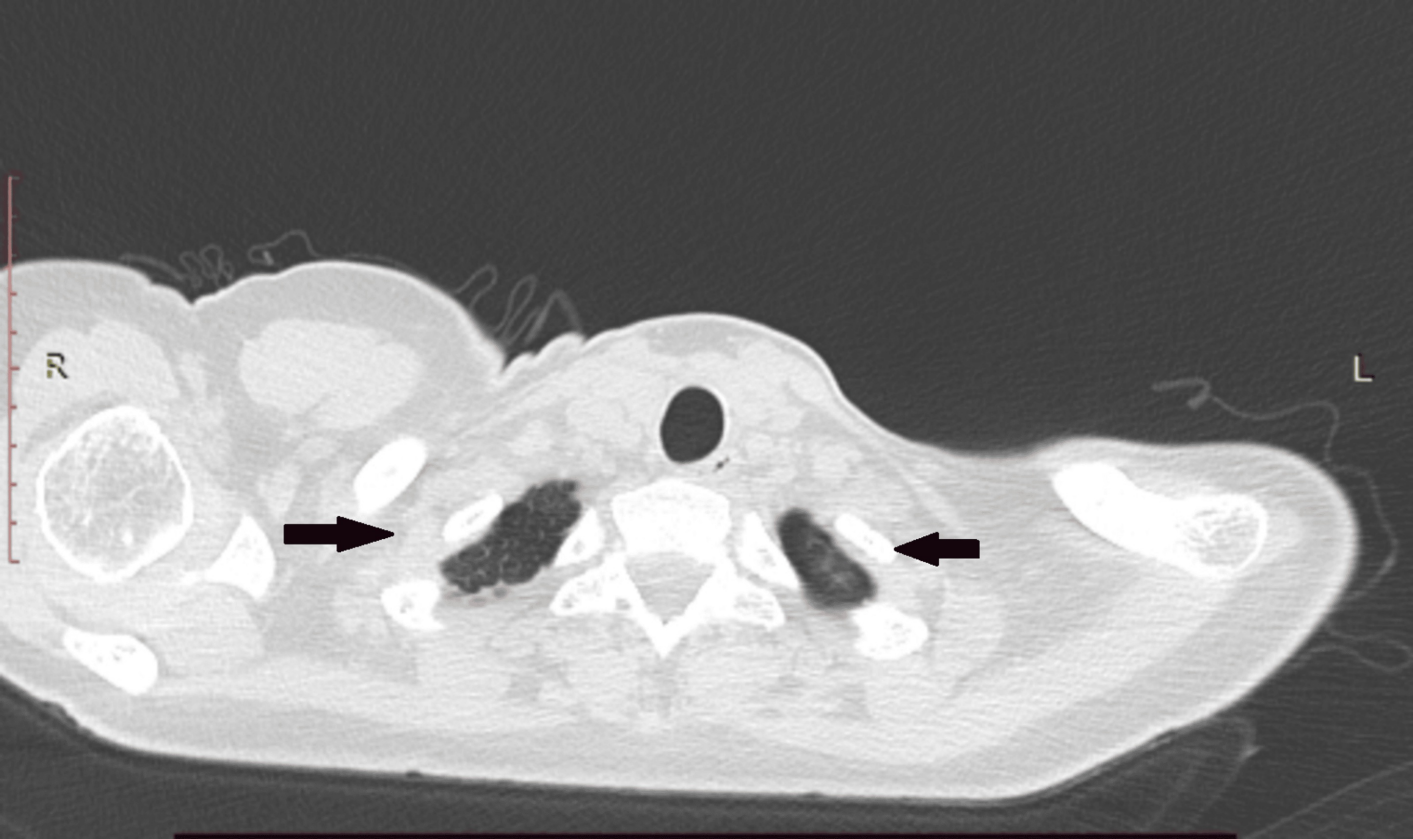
CT revealing multiple bilateral apical lung cysts after the first episode of spontaneous pneumothorax
